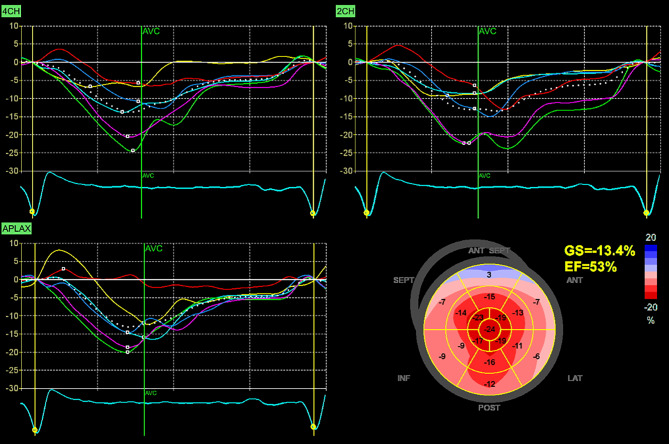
Echocardiography: Two-dimensional strain analysis showing significant reduction of global longitudinal left ventricular strain together with the relative apical sparing pattern
